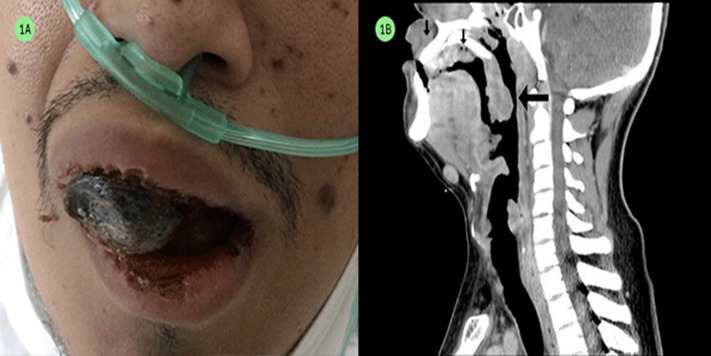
A) mucocutaneous Kaposi sarcoma; B) computed tomography in sagittal plane of oral cavity and the Kaposi sarcoma extension (arrows)
